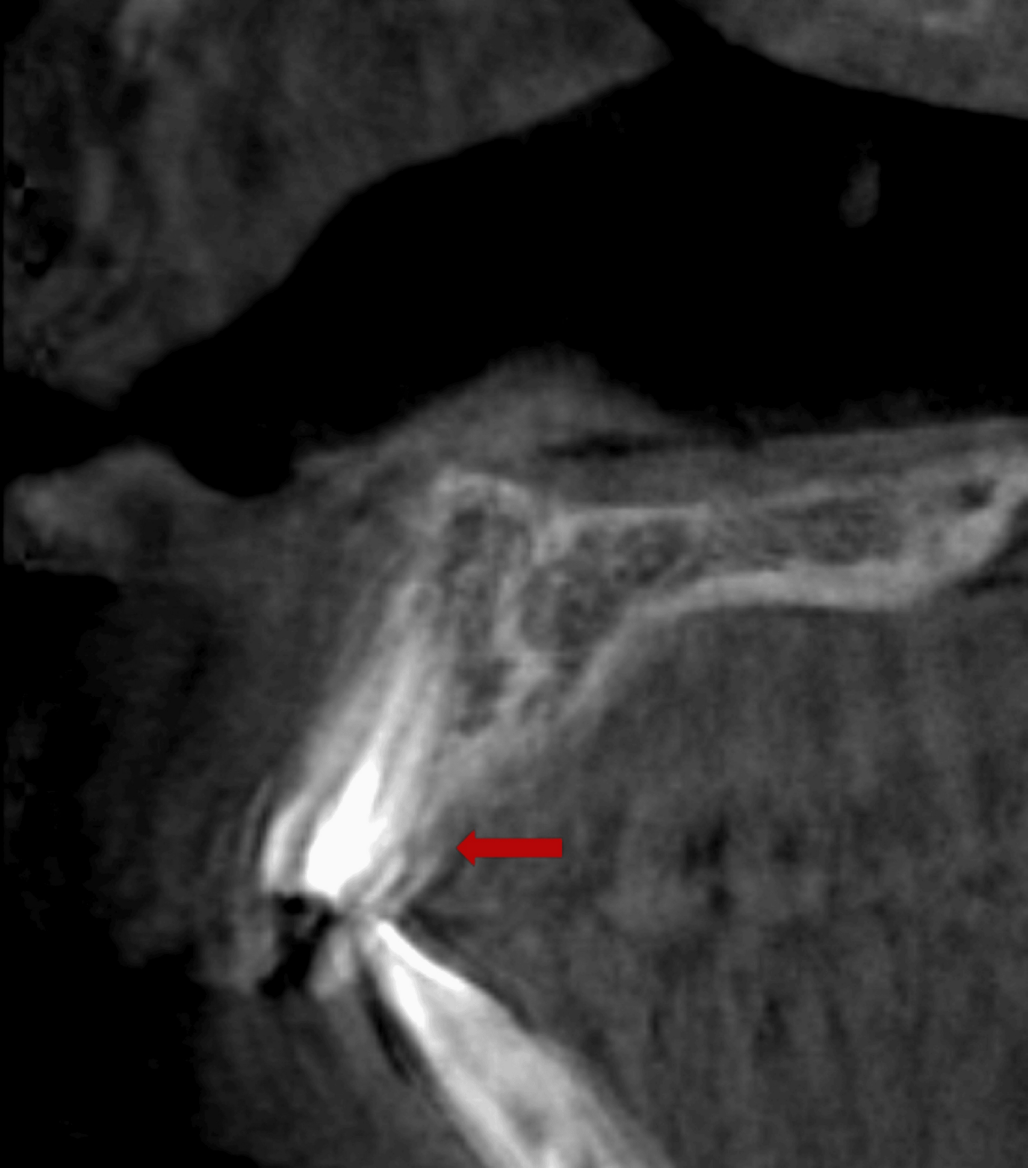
Cone-beam CT (sagittal view) showing the fracture line with overhanging remaining temporary restoration
